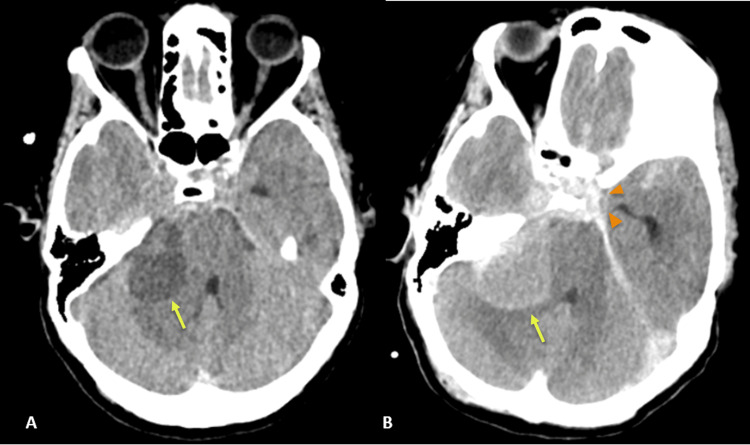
Cranial CT before and after EVD replacement. (A) Cranial CT before EVD replacement showing low density right cerebellopontine angle lesion (yellow arrow); (B) cranial CT after EVD replacement, revealing an increased density of the cerebellopontine lesion (yellow arrow) and additional blood in basal cisterns (orange arrowheads), reflecting re-bleeding.
